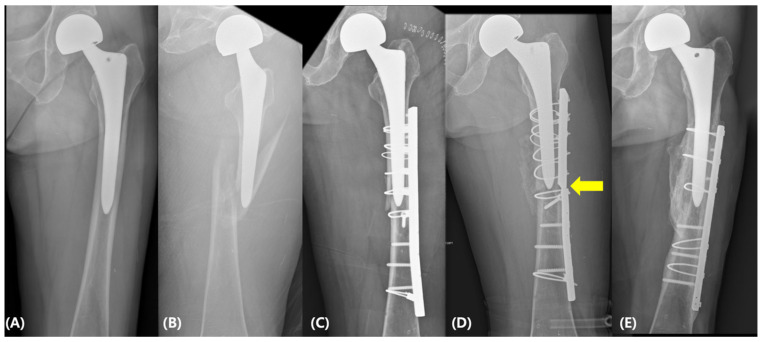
(A) A 77-year-old woman who had previously undergone bipolar hemiarthroplasty for a left femoral neck fracture 4 years earlier. (B) Radiographs obtained after a fall demonstrate a Vancouver type B1 periprosthetic femoral fracture. (C) Open reduction and internal fixation was performed using a non-locking plate with cortical screws and wire fixation. (D) Plate failure (arrow) was identified at 3 months postoperatively, and reoperation was subsequently performed. (E) Radiographs obtained 12 years after the index surgery demonstrate maintained fracture union.
